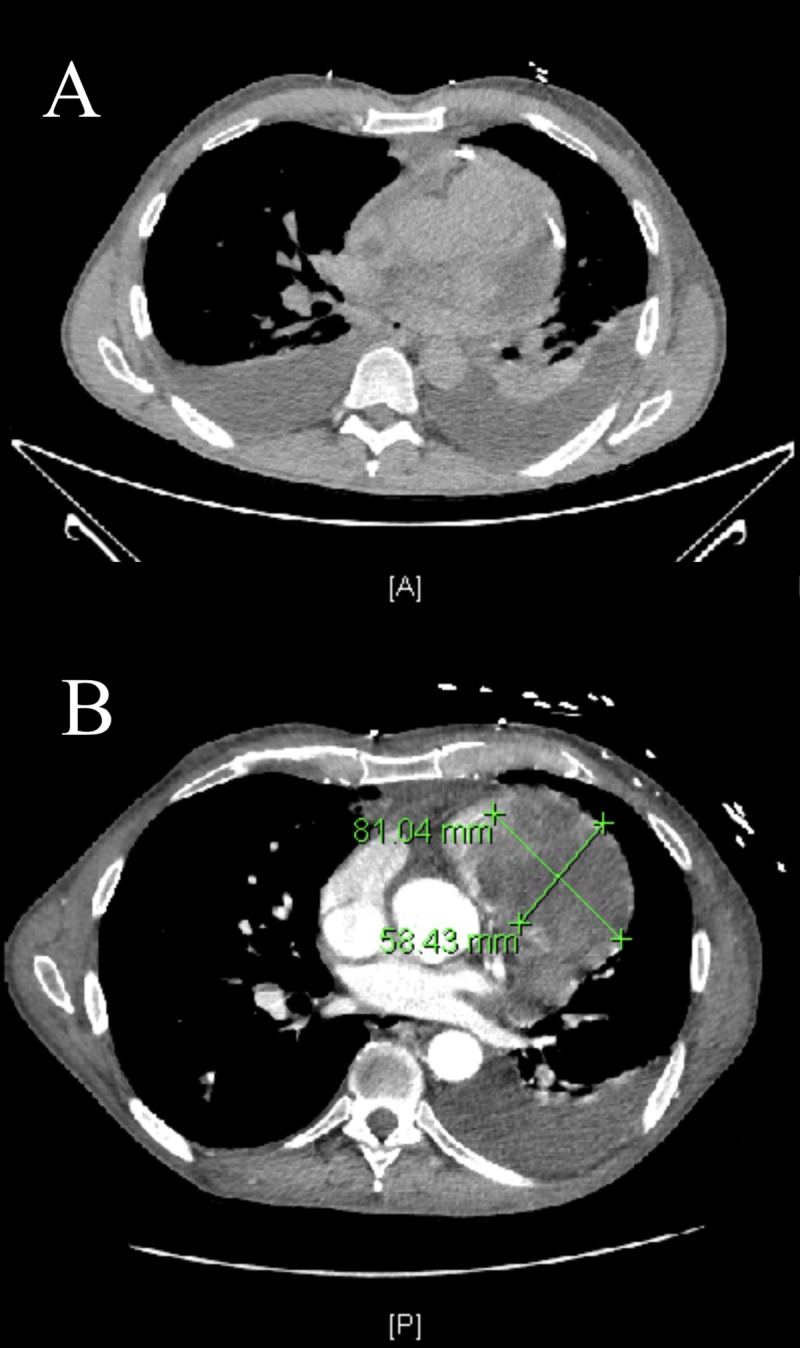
Computed Tomography (CT) Chest Imaging A) Non-contrast CT chest at our institution; B) CT chest with contrast from tertiary care center at a one-week interval showing the development of a large intracardiac mass occupying the right ventricle and the right ventricular outflow tract.
